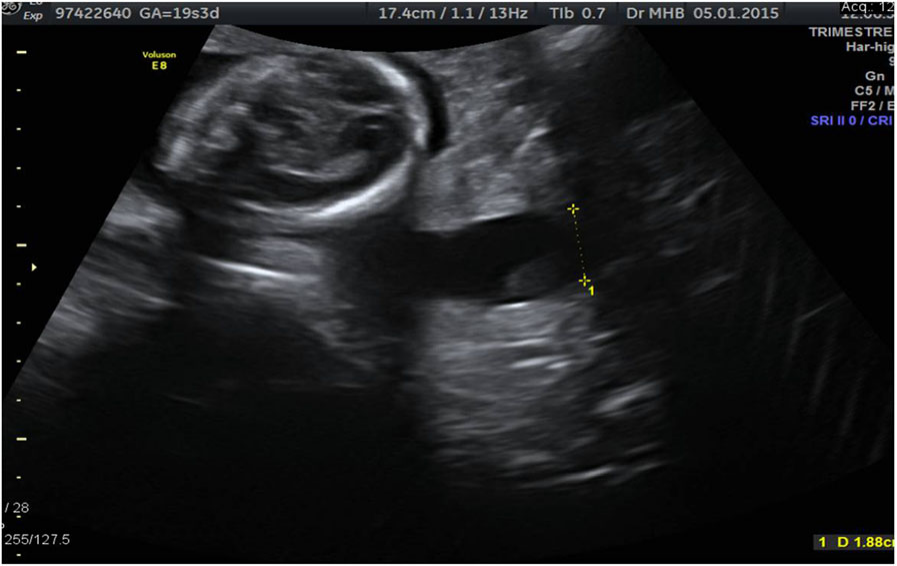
Cervical ultrasound at 19 3/7 weeks showing a long and open cervix
